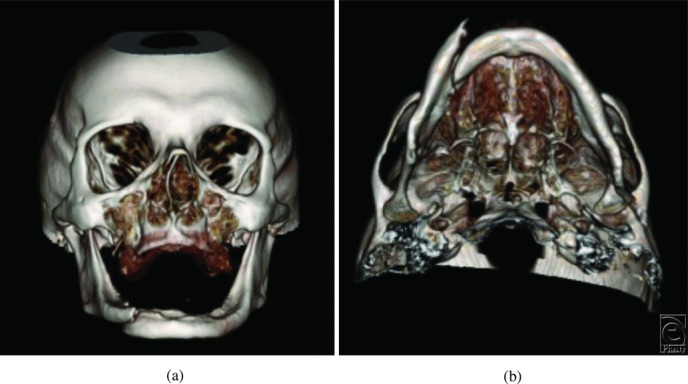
Maxillofacial computed tomography with 3-dimensional reconstruction demonstrating an atrophic, edentulous mandible with fractures of the right body and left subcondylar region.
